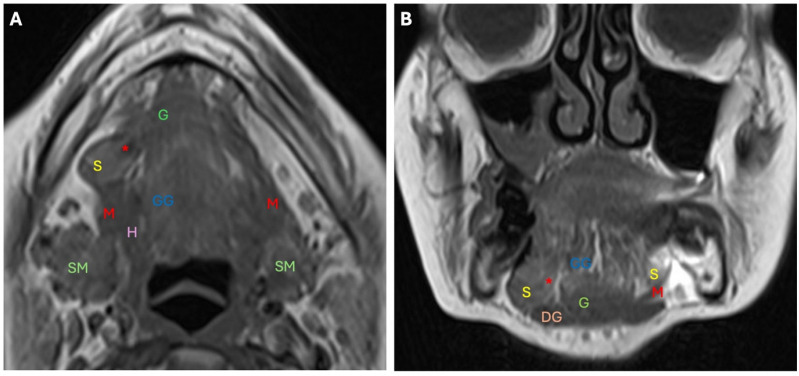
Axial (A) and coronal (B) T1-weighted images show a right mylohyoid boutonniere (*) through which the hypertrophic right sublingual gland (S) herniates into the right submandibular space. S: sublingual gland; SM: submandibular gland; M: mylohyoid muscle; *: boutonniere; G: geniohyoid muscle; GG: genioglossus muscle; H: hyoglossus muscle; DG: anterior belly of digastric muscle.
